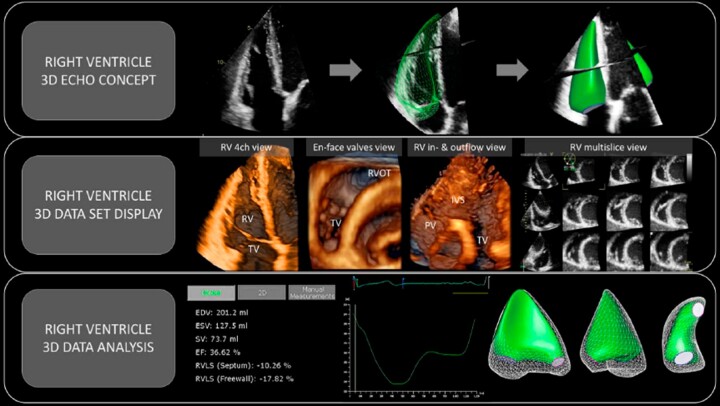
3D echocardiographic assessment of the RV. (*Upper*) Standard 2D echocardiography cannot consider contribution of the RV parts not captured in the scanning plane. 3D echocardiography allows to incorporate all RV components in a single dataset (green surface-rendered model) and enables accurate assessment. (*Middle*) The 3D full-volume dataset of the RV can be cropped, rotated, and displayed in 3D volume rendering mode or multi-slice display, and provide information targeted to exam question (e.g. assessment of RV morphology, valves, RVOT, or interventricular septum). The multi-slice view is useful during data acquisition to ensure that the whole RV is encompassed in the dataset and can also be used for assessment of RV wall motion abnormalities. (*Lower*) Post-processing using dedicated software packages provides RV volumes and EF. It also generates the volume curve which details emptying and filling of the RV during cardiac cycle. Different views of surface-rendered 3D model of the RV enable visual assessment of the RV dynamics and contribution of different components of the RV pump function.
